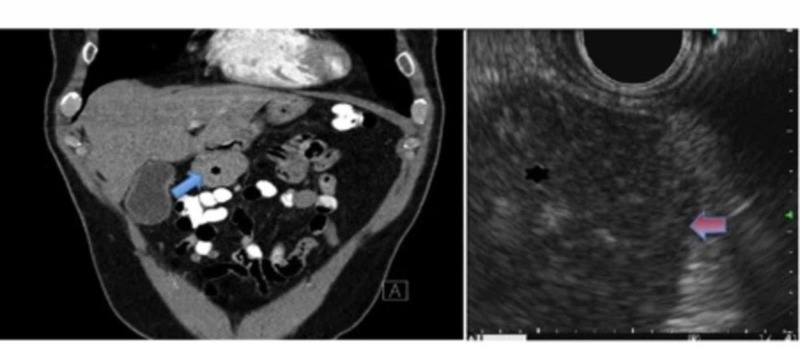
CT abdomen (left image) and EUS (right image) demonstrating GIST EUS: Endoscopic Ultrasound, CT: Computed Tomography, GIST: Gastrointestinal Stromal Tumor
